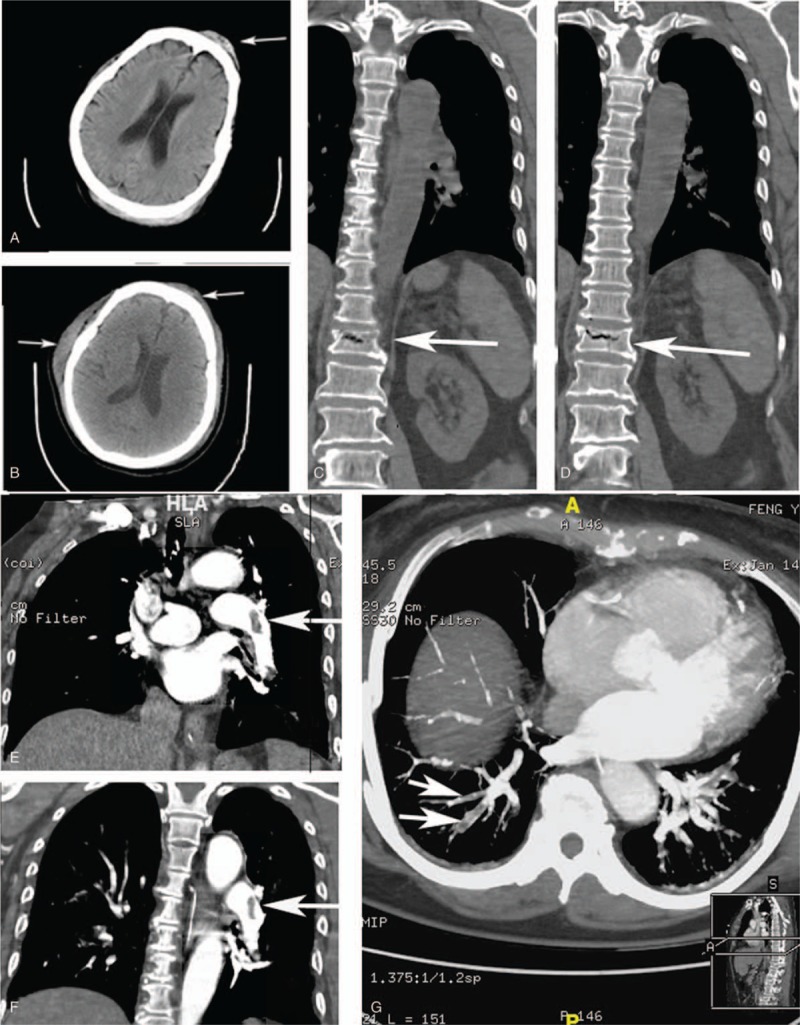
CT image changes in clinical course. (A and B) Head CT scan reveals scalp and frontal hematoma in the first and the third day. (C and D) Thoracic spine CT indicates T12 compression fracture. (E and G) CTPA shows PE with multiple filling defects in the right and left pulmonary arteries. CT = computed tomography, CTPA = computed tomography pulmonary angiography, PE = pulmonary embolism.
